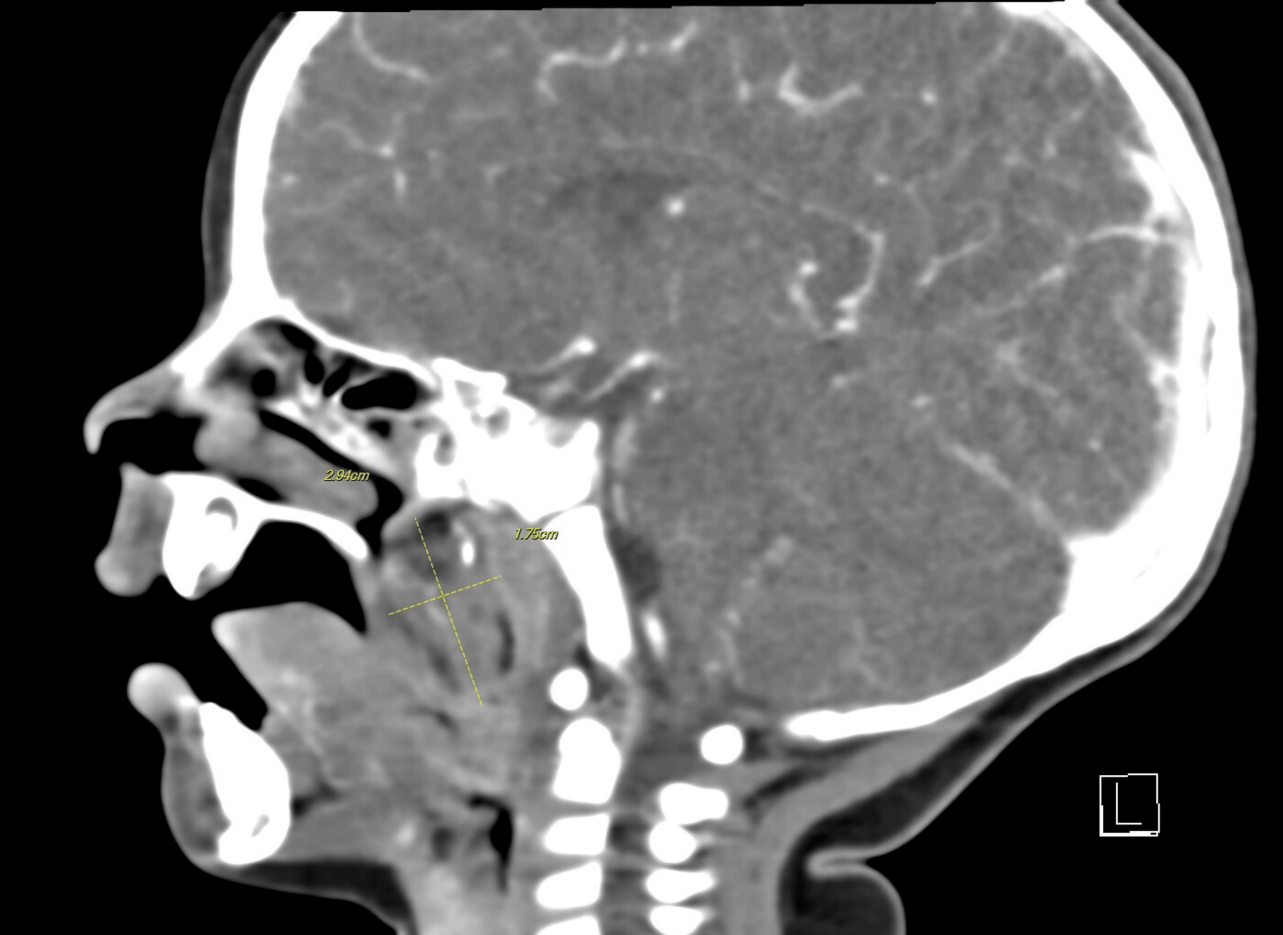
Case 1 CT Preoperative contrast-enhanced sagittal CT showing a midline/right-sided pharyngeal mass with calcifications, fat, soft tissue, and fluid, suggesting a teratoma or epignathus.
